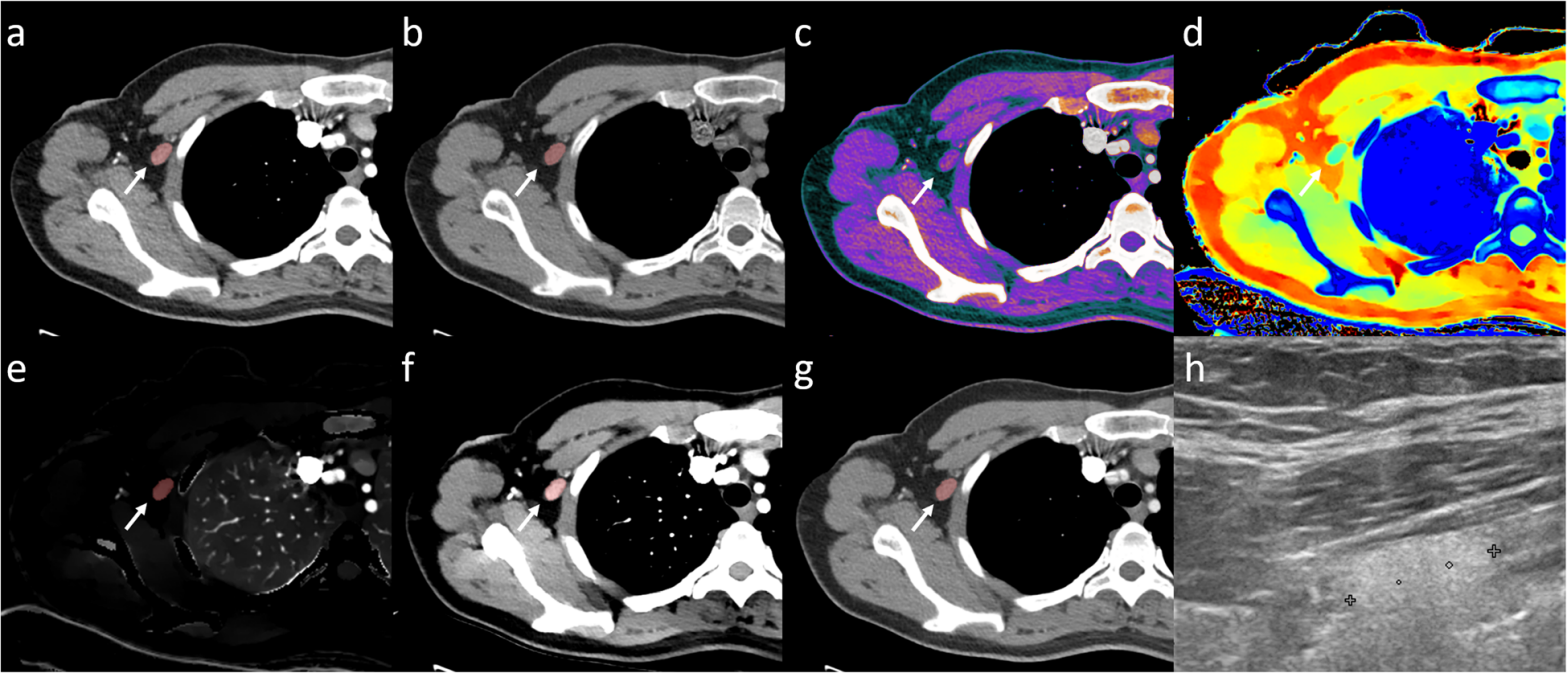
Arterial phase dual-layer spectral computed tomography (DLSCT) examination of a woman with a high attenuating lymph node in the right axilla. The lymph node shows high conventional CT HU (a), Δ contrast enhancement (c) (conventional CT (HU) (a) minus virtual non-contrast (HU) (b)), low Z effective (d), high iodine density (e), and spectral slope ((mono-energy 40 keV (f) − mono-energy 100 keV (g))/60 keV). Based on the cutoff values, DLSCT diagnosed the lymph node as metastatic. Axillary ultrasonography (h) described the lymph node as non-suspicious and consistent with silicone uptake from a previously known breast implant leakage. Sentinel lymph node biopsy was negative for metastatic disease but showed significant silicone content
